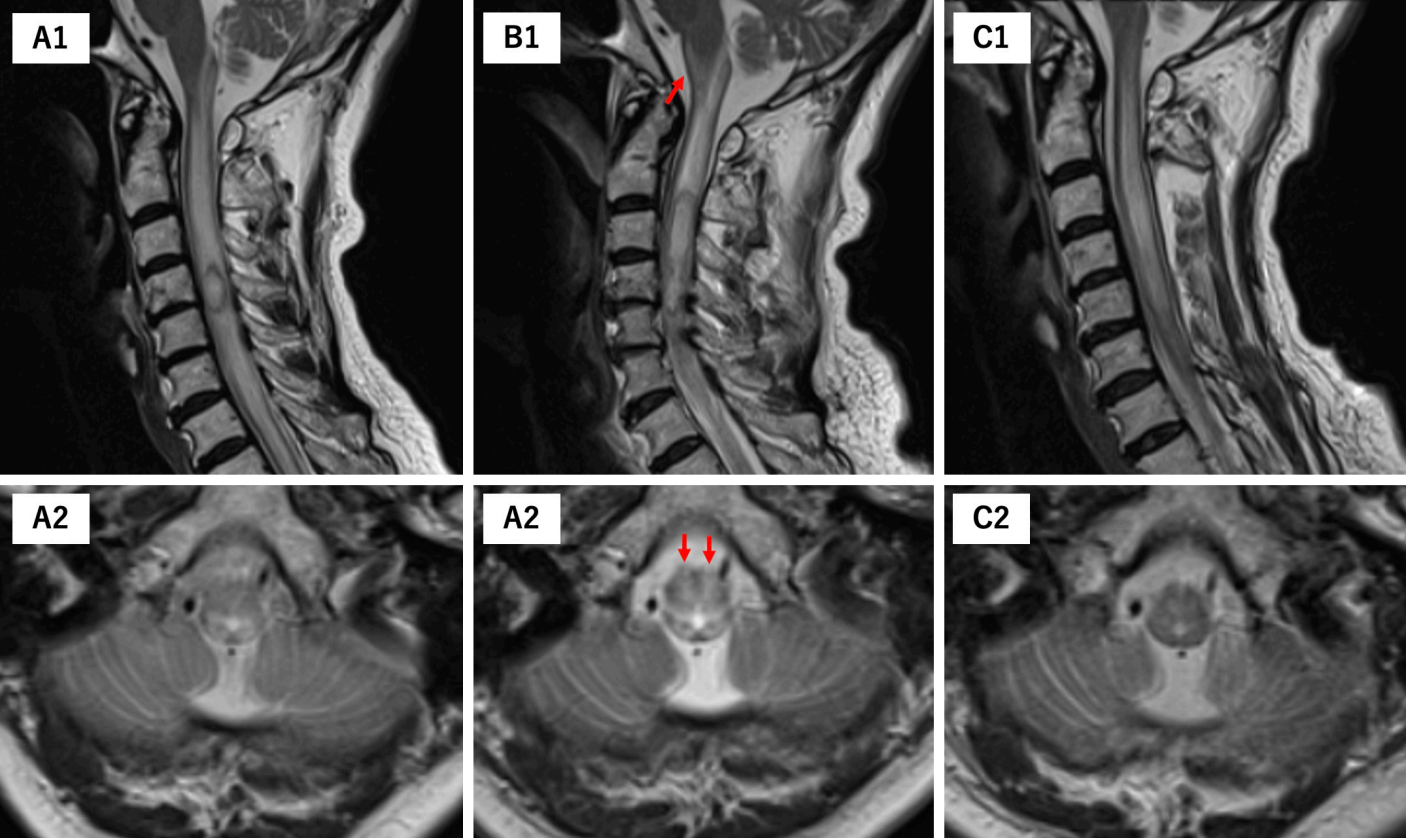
Initial MRI showed edematous changes limited to the dorsal brainstem (A1, 2). When the patient developed impaired consciousness, the MRI revealed that the edema had spread to the central and ventral medulla (red arrows) (B1, 2). Postoperative imaging showed improvement in brainstem edema (C1, 2), which was accompanied by recovery of consciousness. (A1, B1, C1: T2WI Sagittal; A2, B2, C2: T2WI Axial). MRI: magnetic resonance imaging; T2WI: T2 weighted image.
